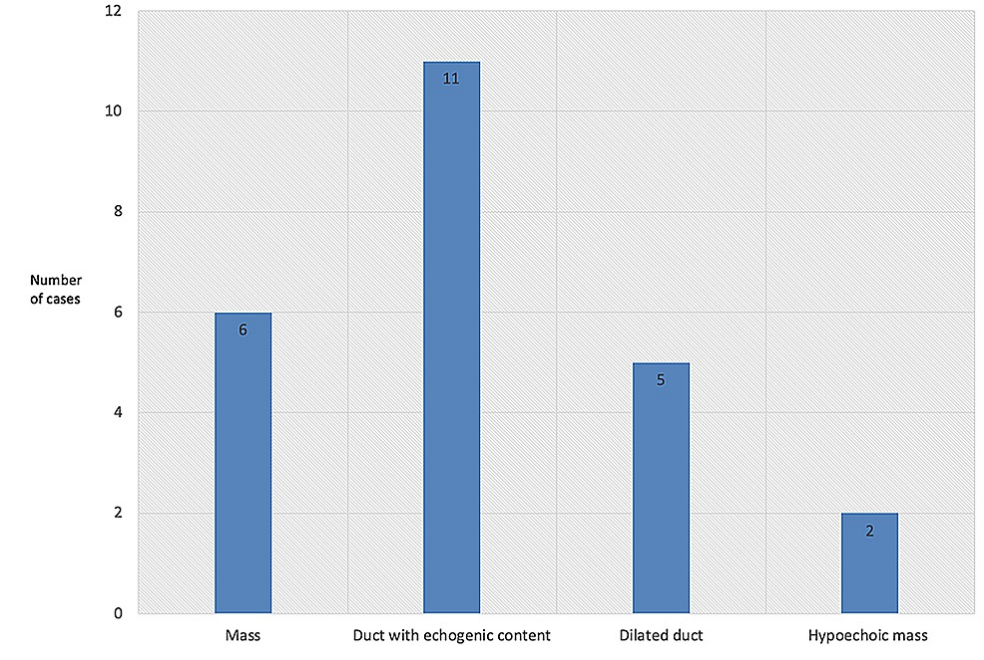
Ultrasound findings of the cases that were radiologically suspected
